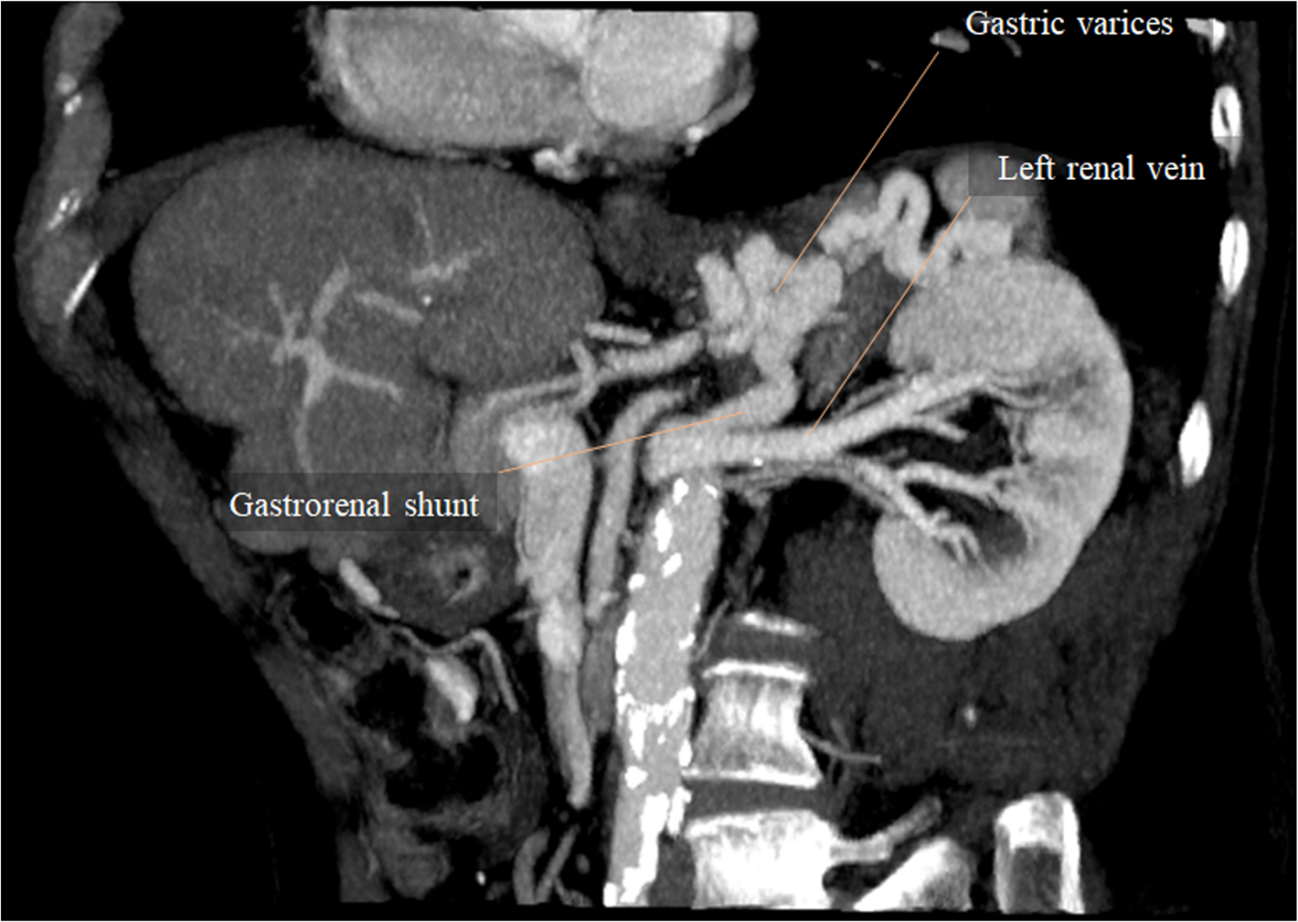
Gastrorenal shunt. MPR image from portal venous phase CT at the level of the left kidney shows a tortuous and high-caliber gastrorenal shunt, running from gastric varices to the left renal vein. Note the increased diameter of the left renal vein downstream shunt confluence, compared to the normal sized left renal vein segment upstream
